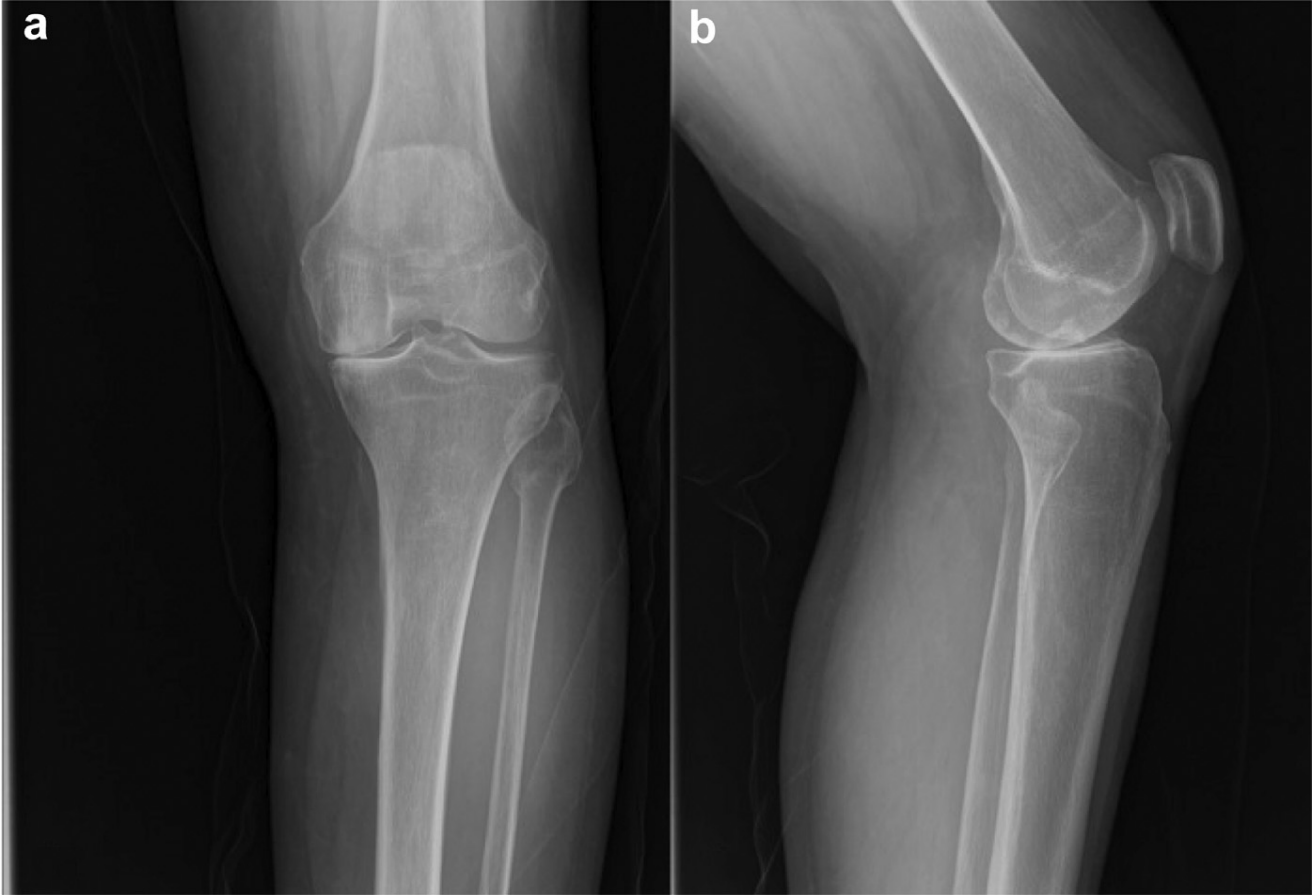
(a) Anteroposterior and (b) lateral radiographs of a study participant showing severe unicompartmental arthritis of the left knee.
